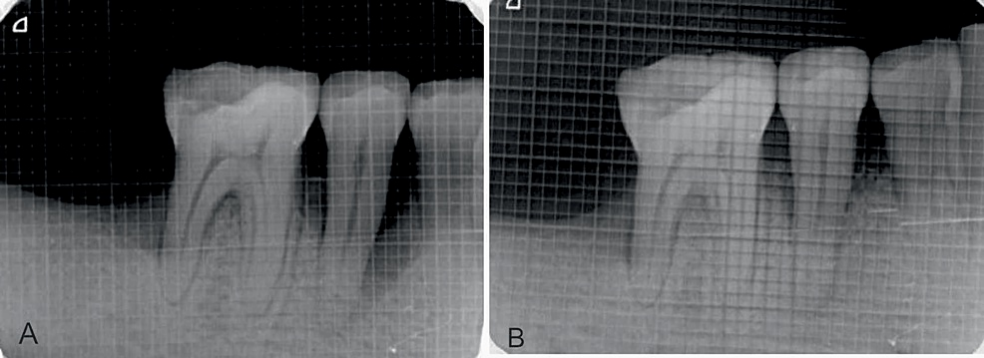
(A) Radiograph shows the baseline bone level at site two (DFDBA). (B) Radiograph showed the bone level at six months at site two (DFDBA). DFDBA: demineralized freeze-dried bone allografts.
